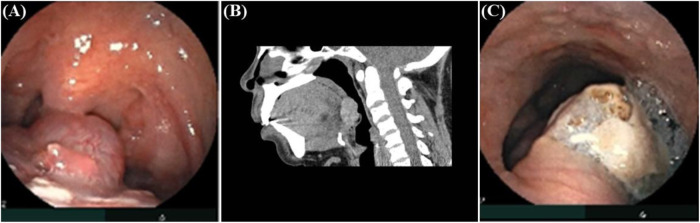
Preoperative and postoperative findings. (A) Preoperative laryngoscopy showing a pale red, smooth-surfaced mass at the tongue base. (B) Sagittal CT scan demonstrating a hyperdense lesion at the tongue base with well-defined margins. (C) Postoperative laryngoscopy on day 4 showing the surgical wound covered with a white pseudomembrane.
